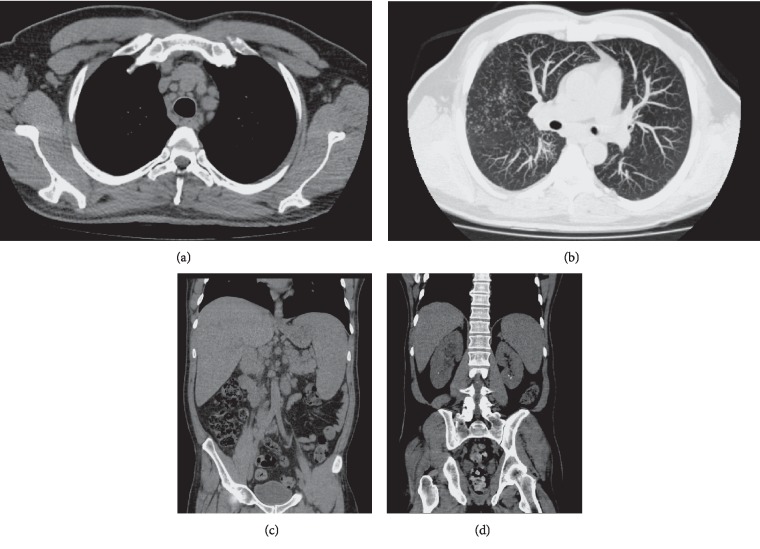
Chest, abdomen, and pelvis computed tomography. (a) Mediastinum with multiple lymph node enlargements in the paratracheal chains. (b) Micronodules in the pulmonary parenchyma with bilateral perilymphatic distribution and enlarged lymph nodes in the pulmonary hilum. (c) Liver and spleen with increased dimensions, and prominent lymph nodes in retroperitoneal and external iliac chains. (d) Kidneys of normal size with nonobstructive calycinal microlithiasis.
